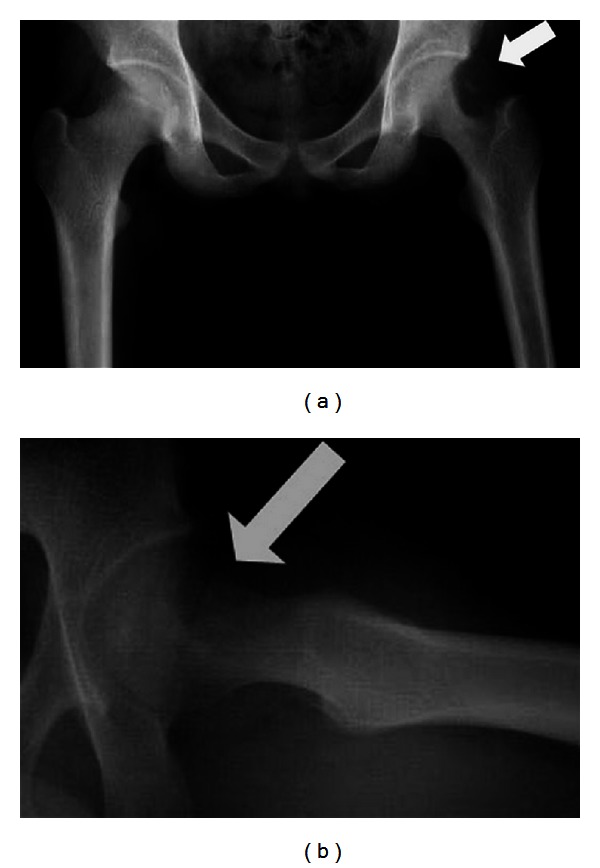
Radiograph showing (a) osteolytic lesion localized to superior aspect of left neck of femur with scalloping medullary margin and (b) the lesion over the anterior aspect of neck extending to head with intact anterior cortex (arrow).
